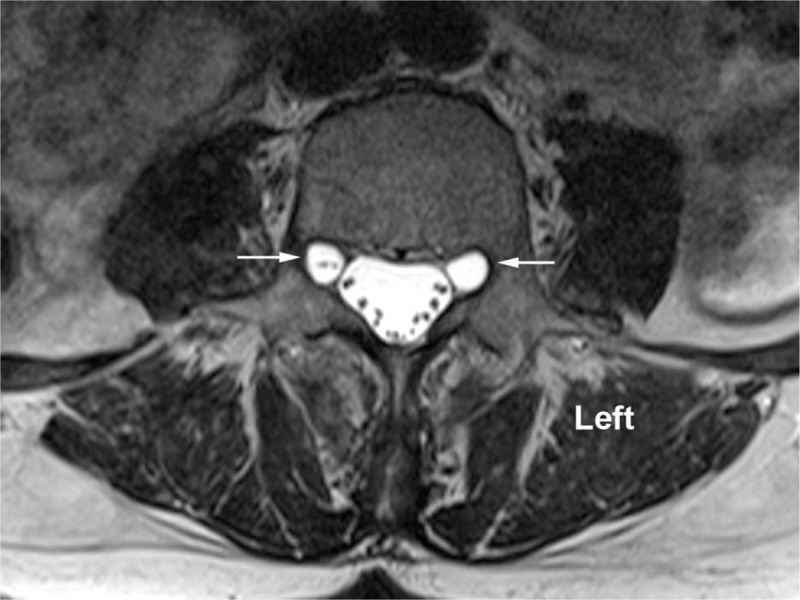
Bilateral foraminal Tarlov cysts at L4–5 level on axial T2-weighted magnetic resonance imaging.
